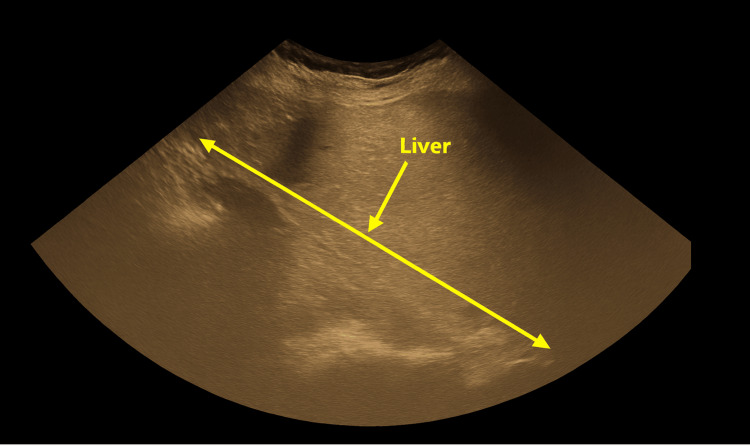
Ultrasound image demonstrating mild hepatomegaly with preserved parenchymal echotexture The long arrow indicates the craniocaudal liver span, and the short arrow marks the hepatic parenchyma, which appears homogeneous without focal lesions. Formal ultrasonography did not reveal any features of biliary obstruction.
